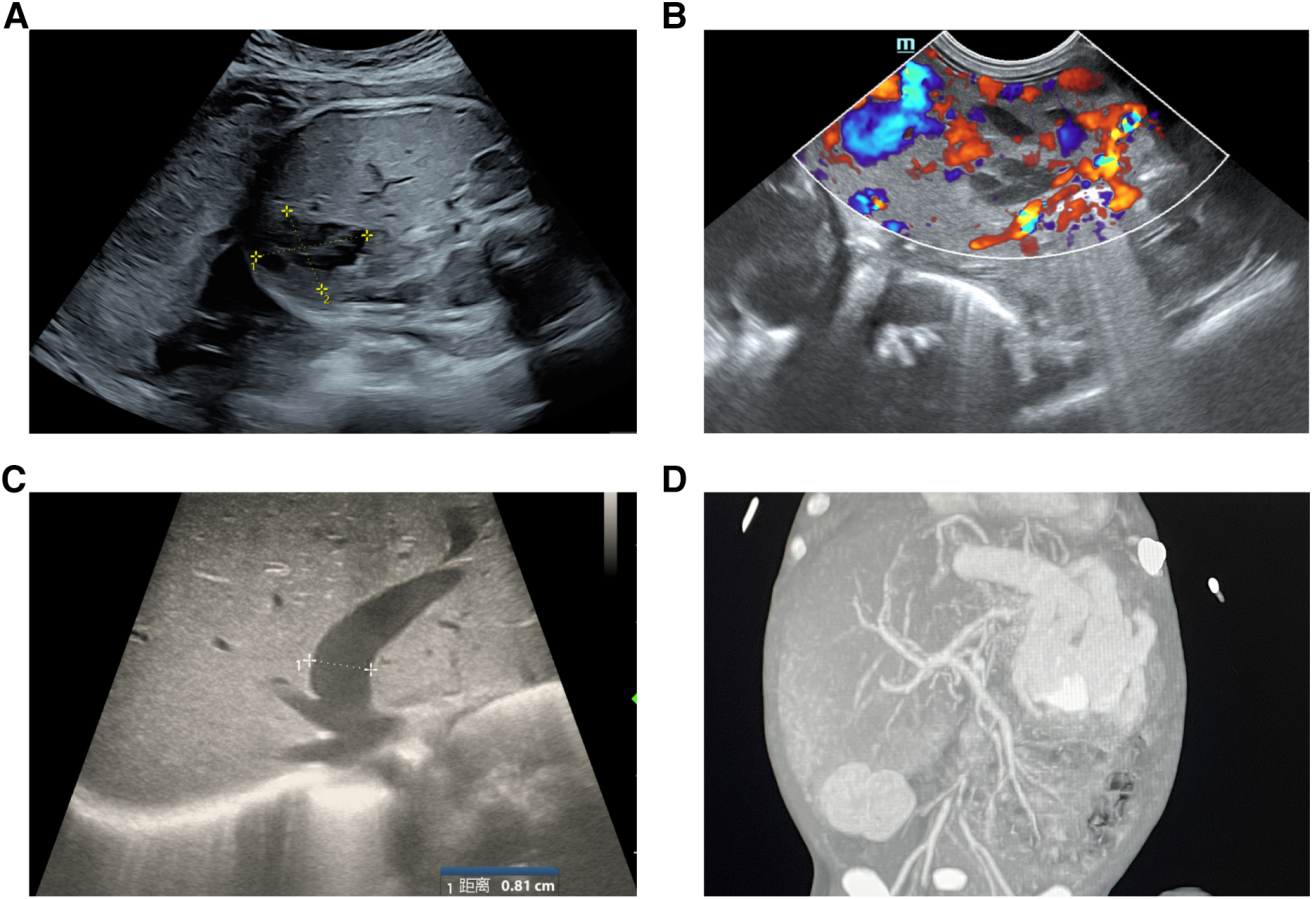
(A) Prenatal ultrasound findings at 28 weeks gestation showed a large, cystic and solid mass (3.6 × 3.6 × 3.0 cm) in the left lobe of the liver. (B) Postpartum hepatic ultrasonography showed a 5.3 × 4.7 × 3.3 cm mass with abundant blood signal. (C)The diameter of the left hepatic vein was dilated (0.8 cm). (D) Enhanced CT revealed a large arteriovenous fistula in the left hepatic lobe.
